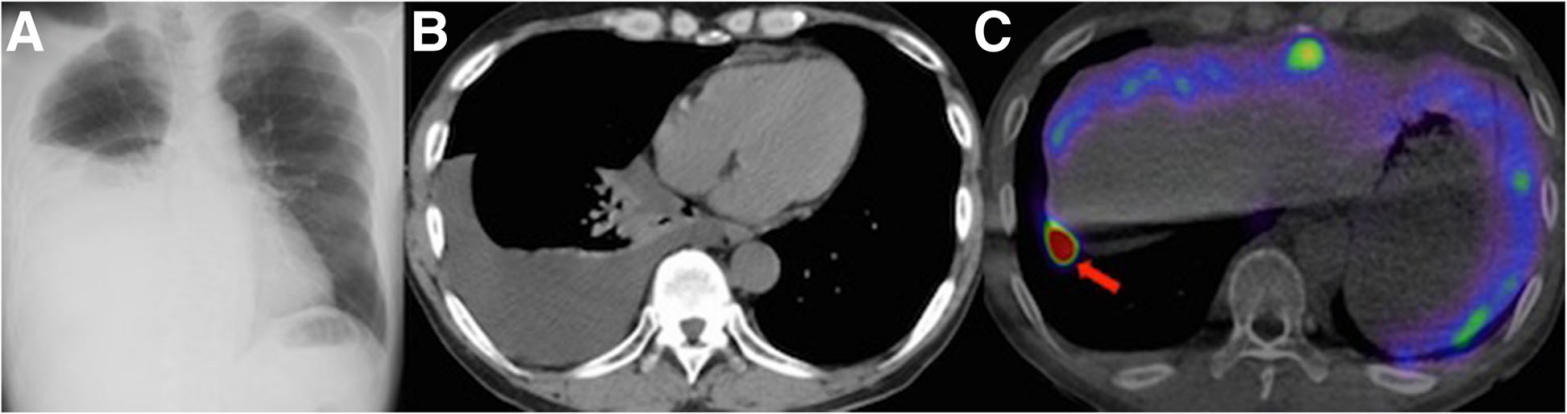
Imaging findings. a Chest X-ray radiogram of 65-year-old man with chronic renal failure. Chest X-ray showed a right hydrothorax. b Chest computed tomogram (CT) of the patient. Chest CT showed hydrothorax in the right thoracic cavity. c Chest ^99m^Tc-macro-aggregated albumin (^99m^Tc-MAA) radioscintigraphic image of the patient. ^99m^Tc-MAA was administered into the peritoneal cavity with dialysate, and 450 min later, a leakage of the dialysate into the right pleural cavity was detected (red arrow)
